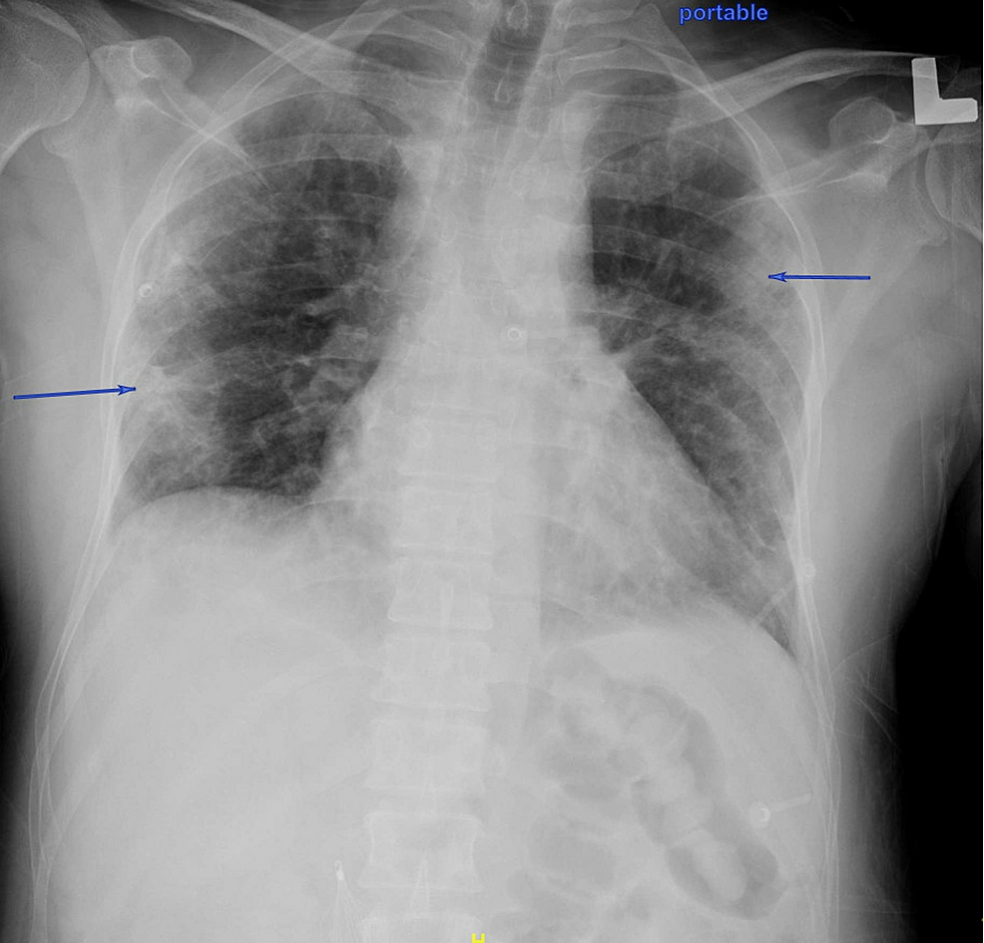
Chest X-ray at presentation Blue arrows: Peripheral bilateral patchy opacities
